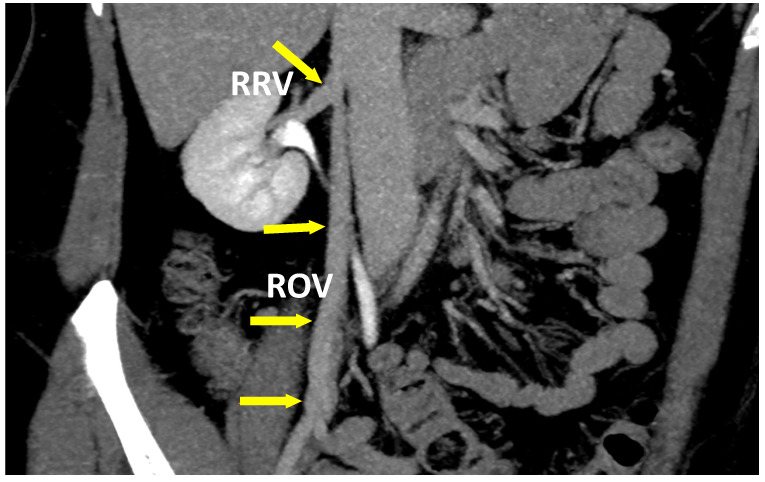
The coronal plane of a CT-V scan in a patient with atypical ROV outflow. Blood from ROV is drained into RRV.
